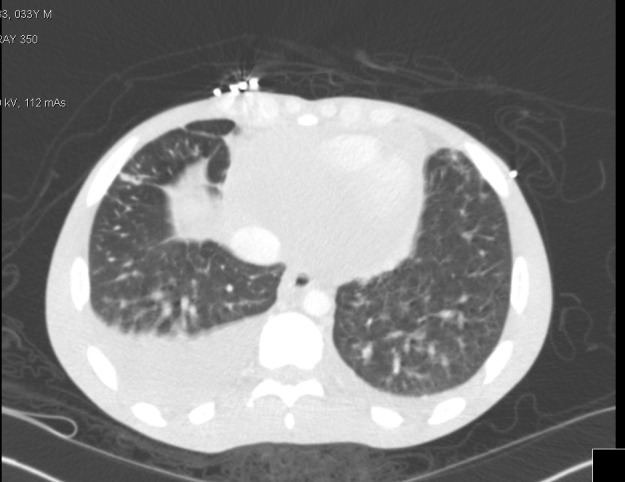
CT chest with contrast revealed the presence of peribronchovascular airspace consolidation in bilateral upper lobes with intrinsic cavities in association with bilateral pleural effusion (greater on the right)
